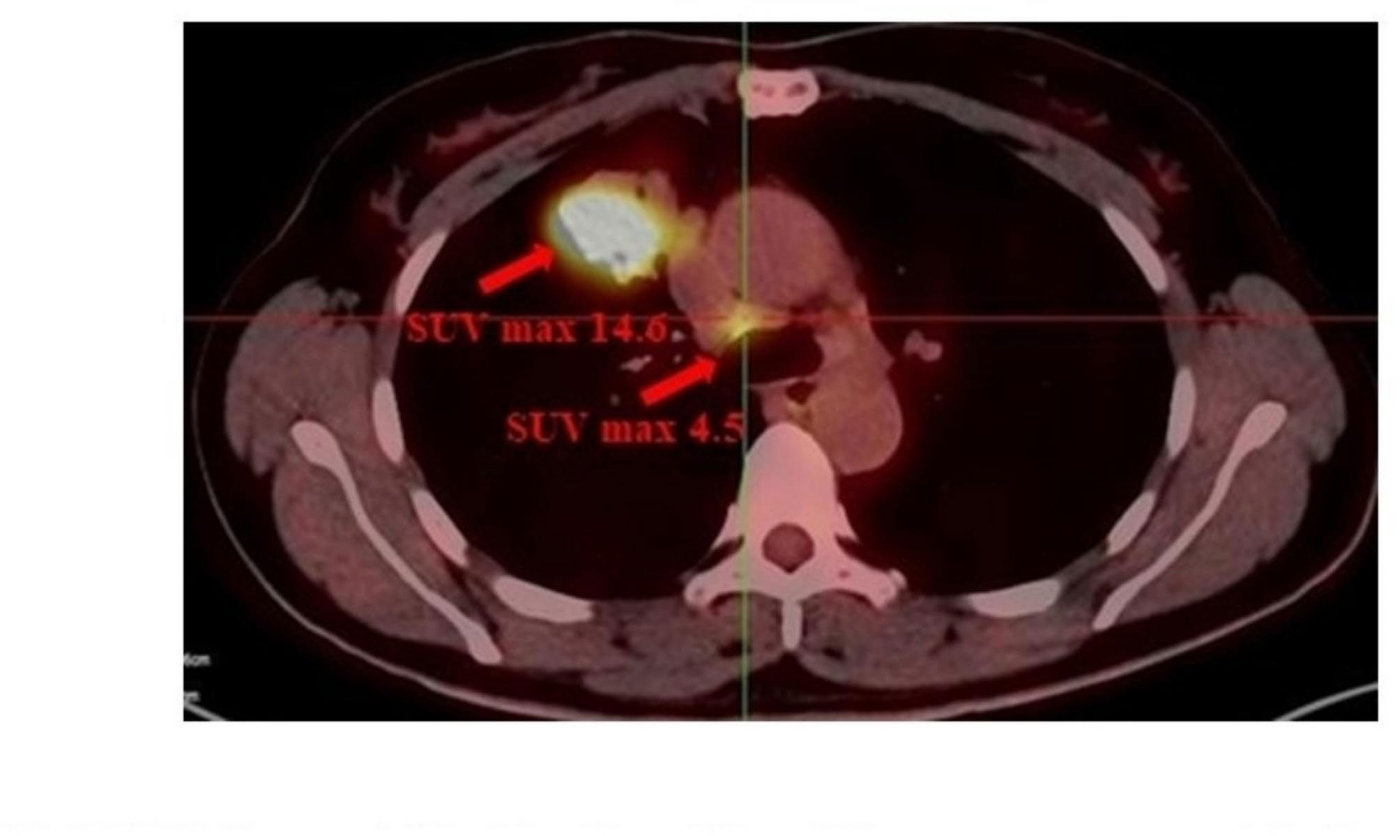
PET-CT suggested that the 40mm*39mm*34mm mass was suspected of lung cancer with SUV max 14.6 and the swollen mediastinal lymph node 4R with approximately 10mm short axis had a high uptake (SUV max = 4.5)
